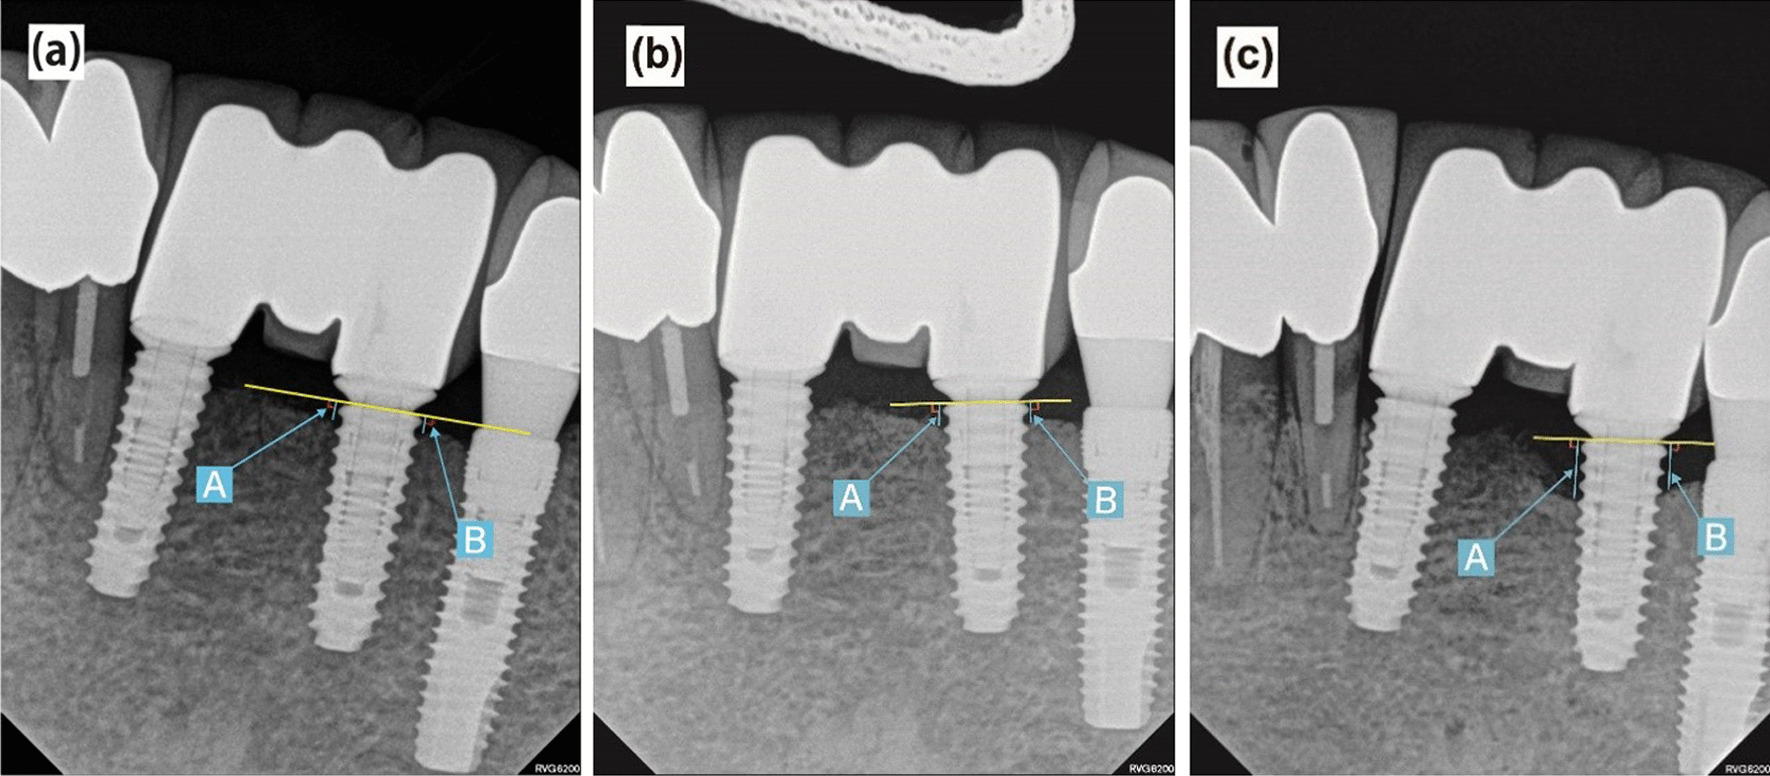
Landmarks of radiographic measurements. a Immediately after the prosthesis was installed; b Periapical view at 1-year after prosthetic loading; c Final periapical radiograph; A Shoulder to mesial contact point (mm); B Shoulder to distal contact point (mm); The average of A and B was the amount of marginal bone loss
